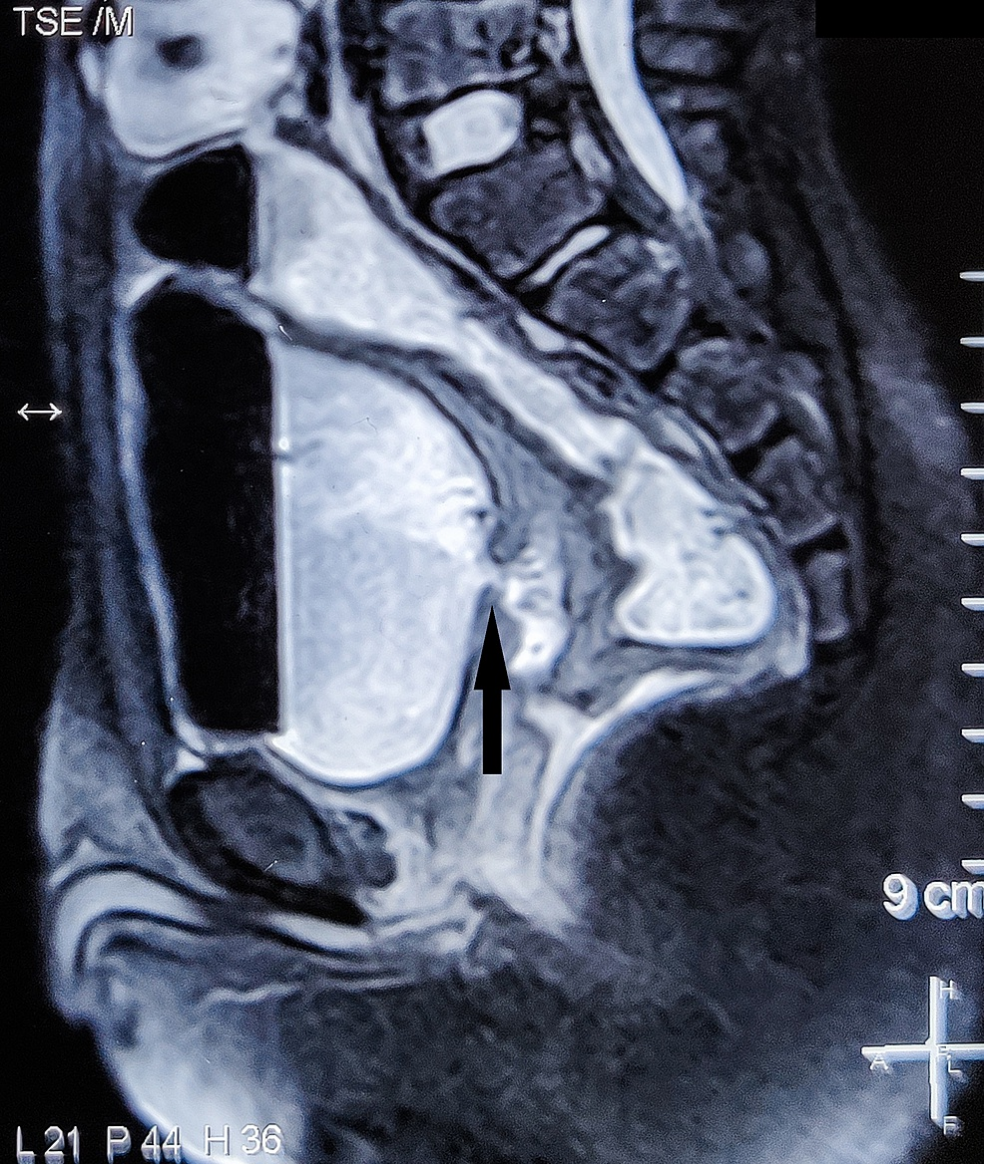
Saggital section of MRI of the pelvis showing the fistulous tract (arrow)
